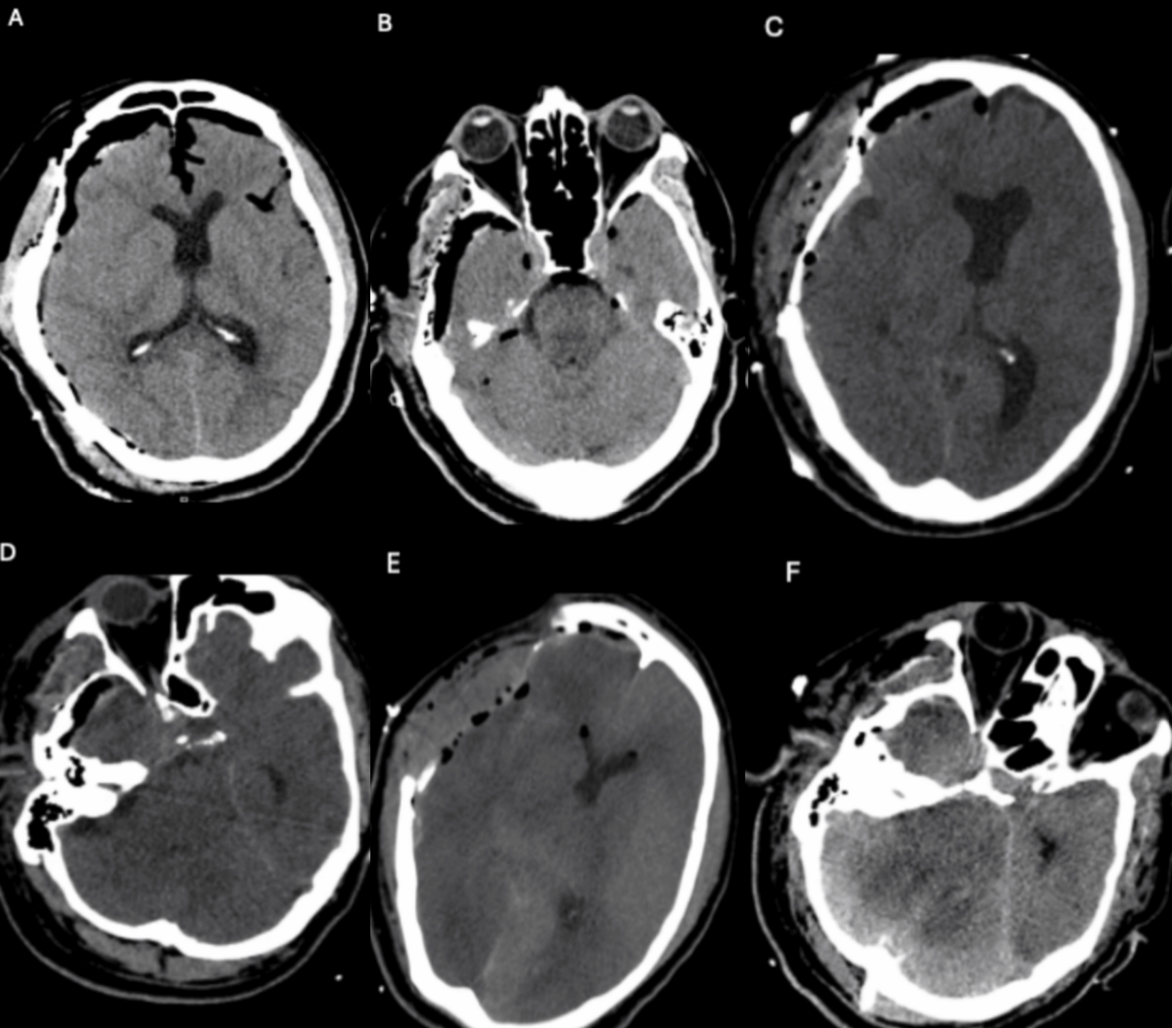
Post-operative and Interval Head CTs A, B: Initial post operative CT head reveals good decompression with no midline shift and pneumocephalus present within the areas of cisternostomy C, D: Interval CT scan the next day at time of patient clinical decline reveals recurring midline shift out of proportion with evidence of brain sag and crowding
